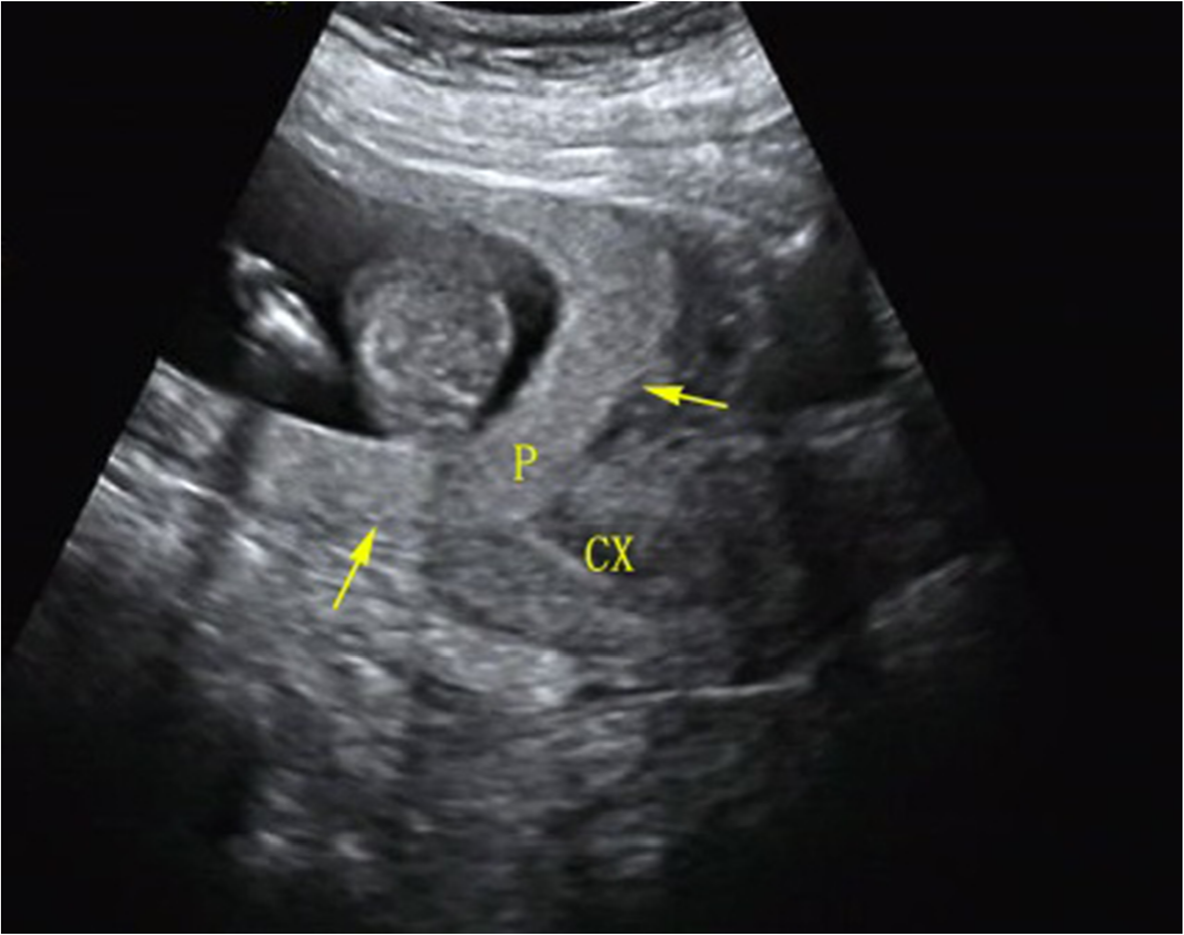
Heterotopic complete placenta previa and placenta accreta at ultrasonography at 16+3 weeks’ gestation
